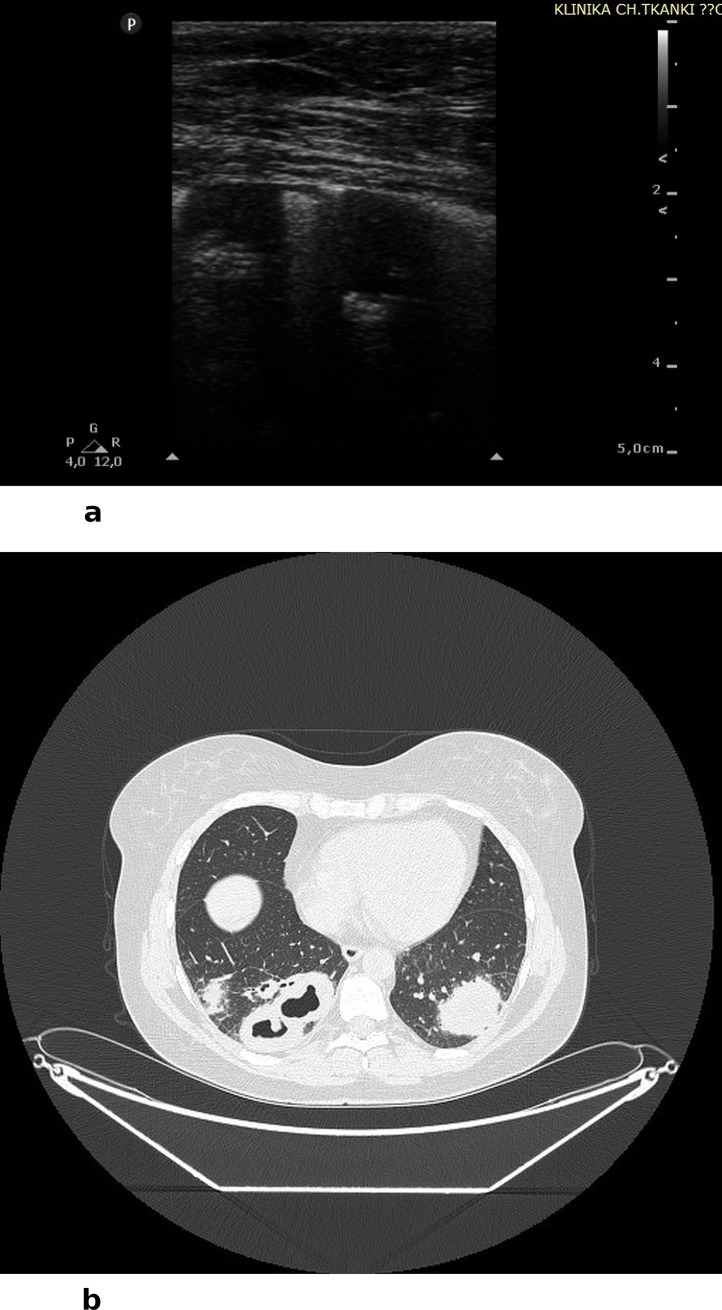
A) LUS: two round hypoechoic consolidations (infiltrates), partly disintegrated, hypoechoic pulmonary pleura, B) CT: Subpleural round lesions with features of disintegration, creating caves.
